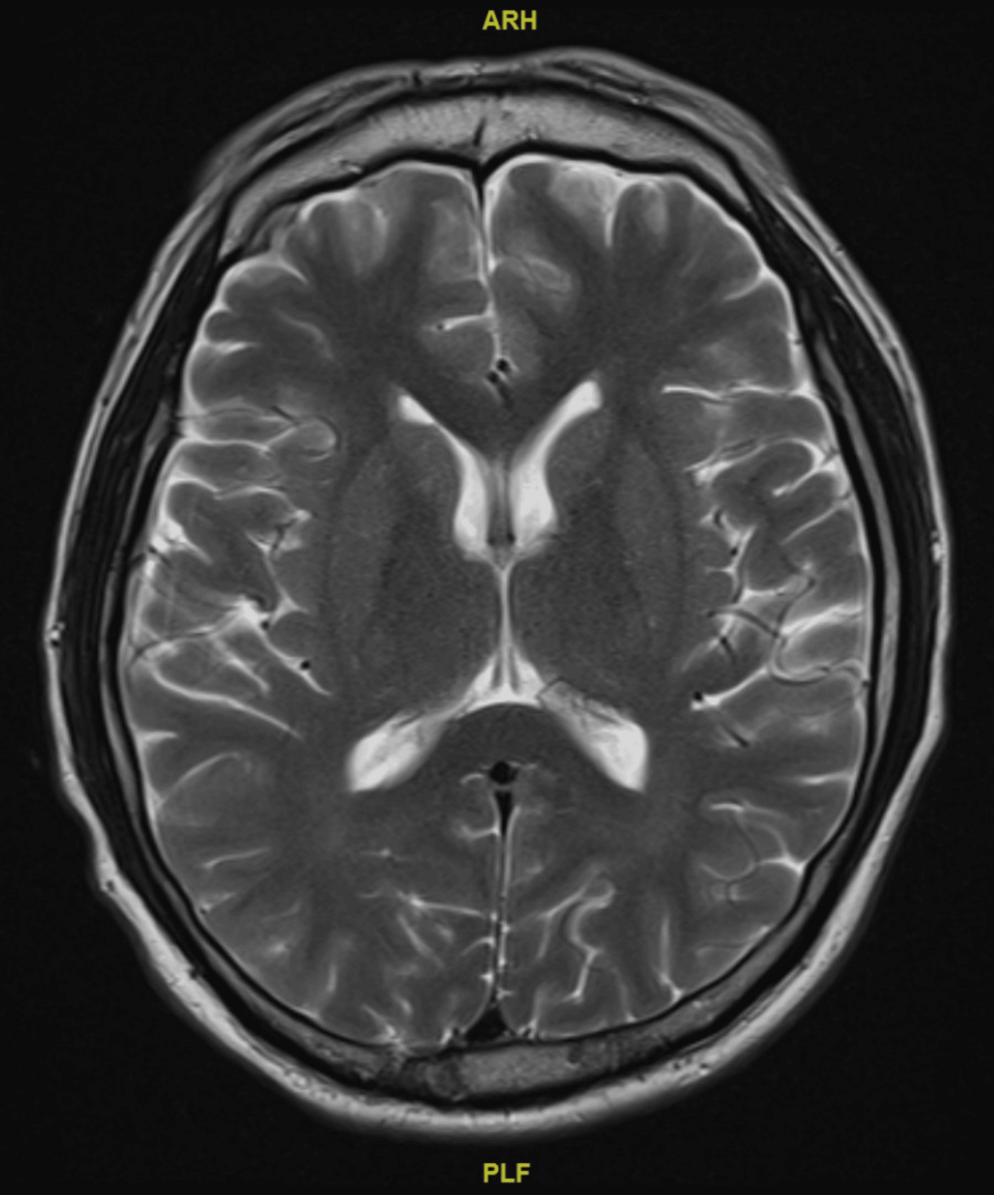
Non-contrast magnetic resonance imaging of the patient's brain, axial section Permission to use the above brain image for the purpose of this case report was obtained from Ng Teng Fong General Hospital.
